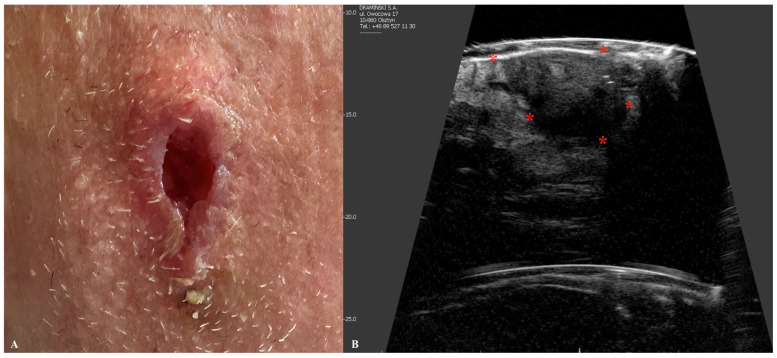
Macroscopic image of SCC (A) with a corresponding ultrasonographic image (B) showing a hypoechoic infiltrative nodule with irregular, ill-defined borders. Stars indicate tumor margins.
